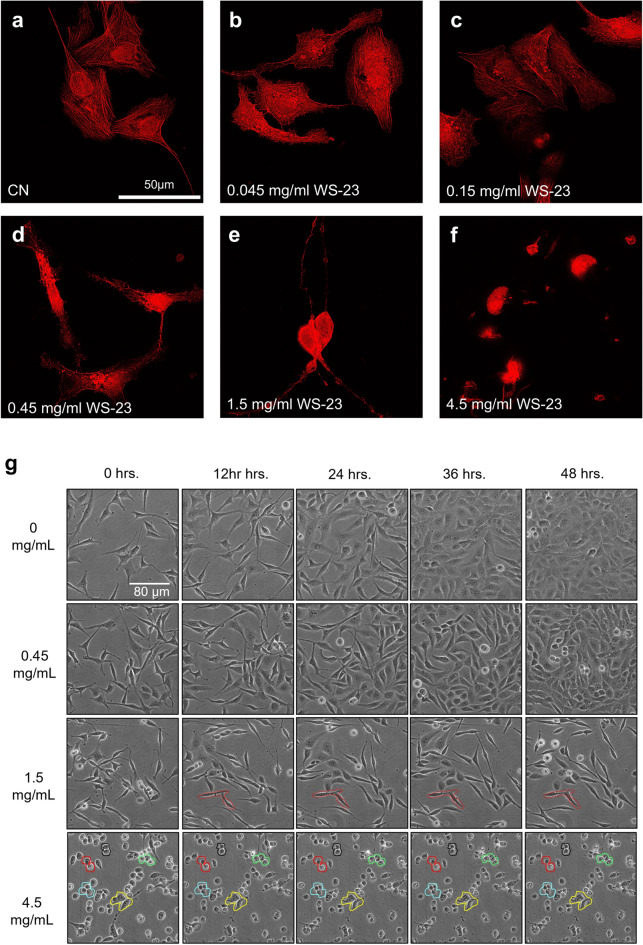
BEAS-2B cells labeled with phalloidin following WS-23 treatment in submerged culture. (a–f) Fluorescent images of cells treated with varying concentrations of WS-23 then labeled with phalloidin. (g) Live cell images captured in a BioStation CT during treatment with WS-23. Cells circled in colors did not move during the treatment period. Concentrations are those present in the e-liquid before aerosolization.
